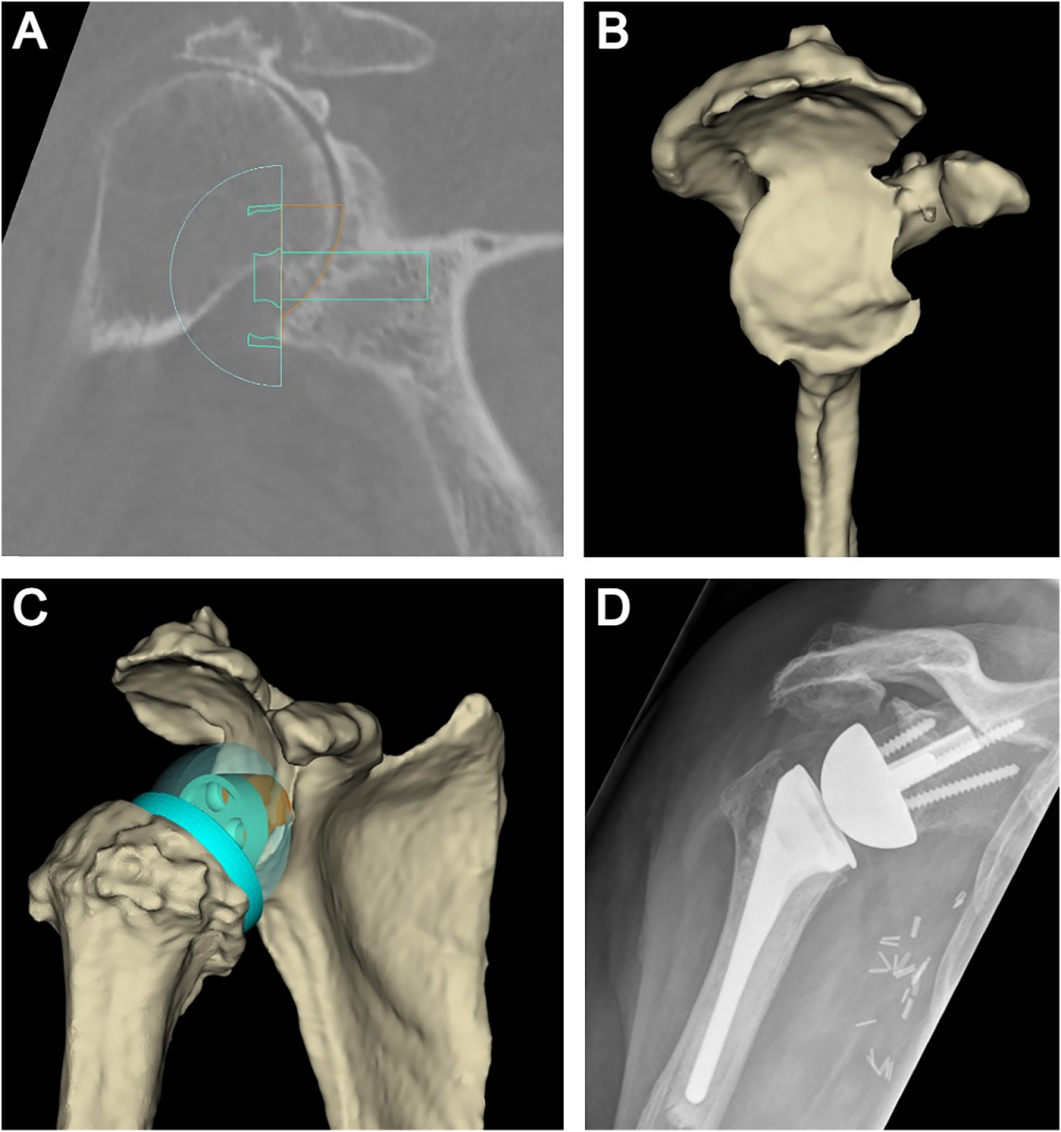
(A) 3D planning software in the coronal plane is used to determine the optimal position of the glenoid baseplate to correct glenoid inclination and avoid superior tilt. (B) 3D rendering with subtraction of the humeral head reveals the primary glenoid deformity in the posterior-superior quadrant (Favard Type E3 glenoid) in this case example. (C) Patient-specific bone graft (ie, digital graft) that is created using the planning software to address the glenoid deformity. (D) Postoperative anteroposterior radiograph of the shoulder revealing the patient-specific bone graft with correction of glenoid inclination.
